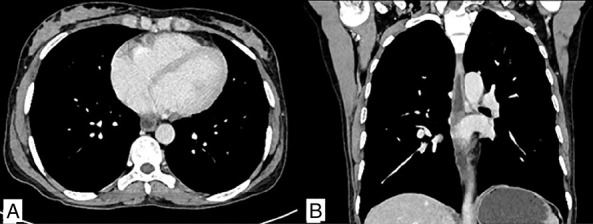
Contrast-enhanced computed tomography of the thorax. (A and B) Axial and coronal images showing no abnormal findings.
